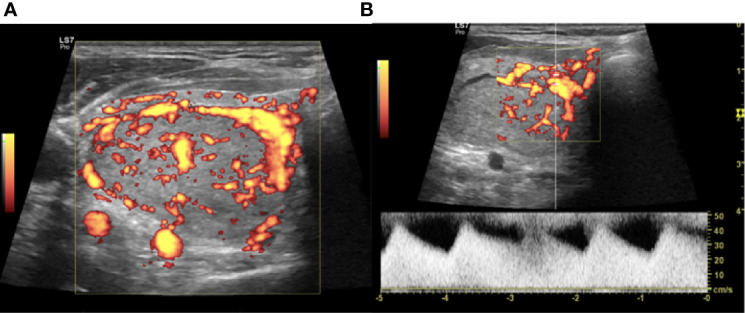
Right lobe nodule, intraoperative transverse ultrasound (US) scan. (A) Power Doppler (PD) evaluation of the nodule to be treated with vascular radiofrequency ablation (vRFA). (B) Identification at PD and PWD of the main afferent artery.
